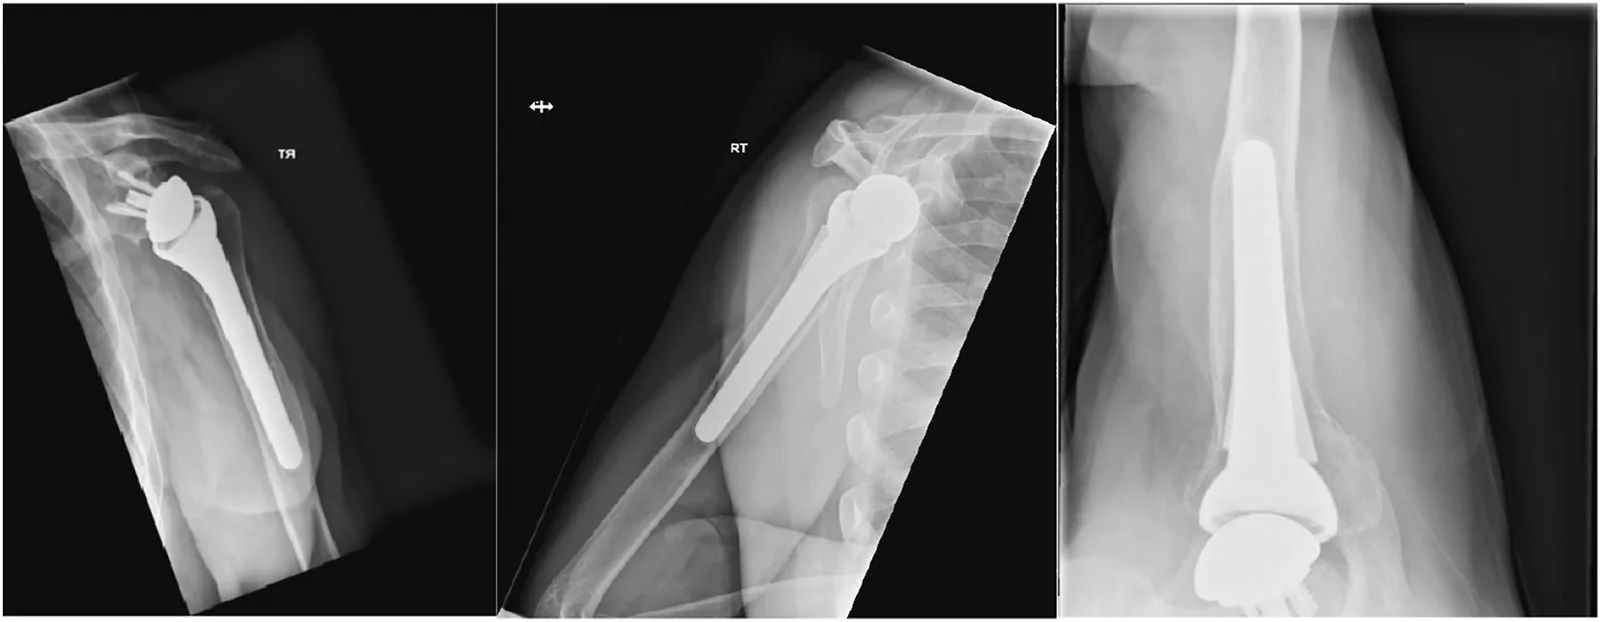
Case 1 x-rays at a 2-year follow-up visit.
